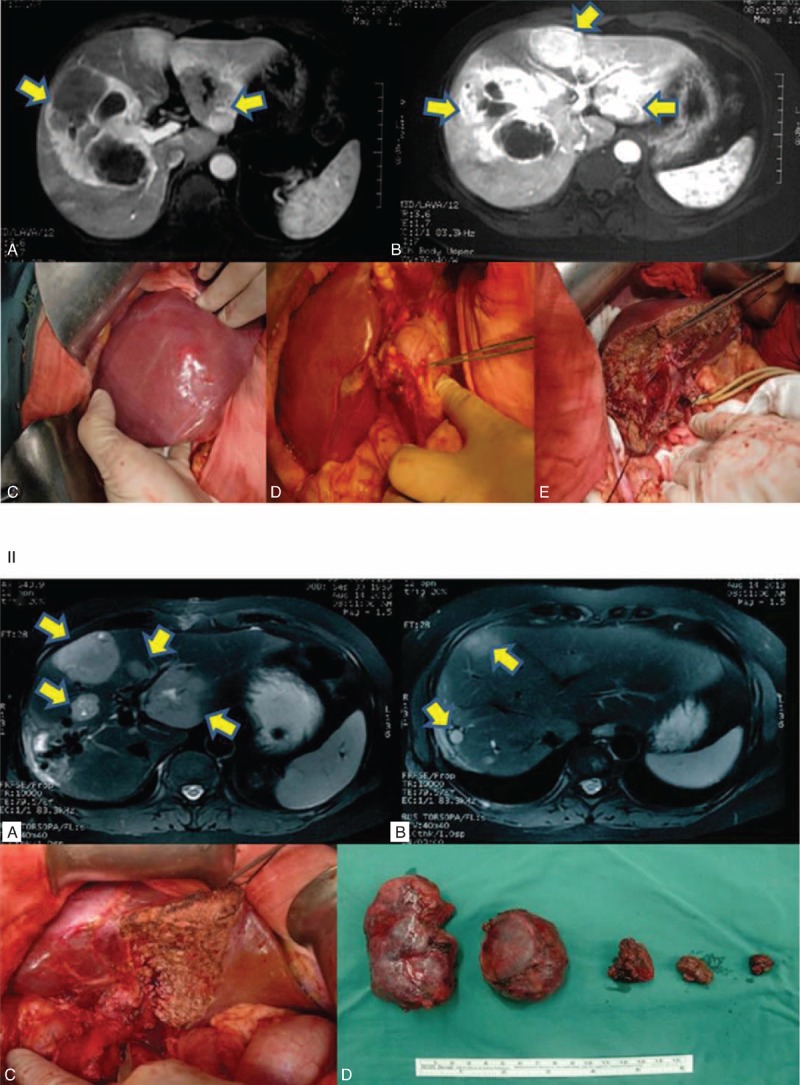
MRI and surgical data on 1 patient with G2-level NELM. A 52-year-old female patient was diagnosed with NELM with 6 liver neoplasms totaling 31 cm in size. Two separate surgical resections were performed. The first resection removed the largest tumor of 15 cm. The liver was allowed to regenerate to sufficient volume to sustain secondary resection. All 5 other neoplasms were removed during the secondary resection, which was performed 4 months after the first one. The patient was in good condition at the time of the preparation of this manuscript. Panel I, MRI images and key steps of first resection. (A & B) MRI images, arrows indicate locations of tumors; (C) a 15-cm tumor was found that covers sections of V, VI, VII, and VIII of liver; (D) primary tumor was found on the lesser curvature of the stomach; (E) remnant tissue of right lobe after resection. Panel II, MRI images and photos of second resection. (A & B) MRI images, arrows indicate locations of tumors; (C) remnant tissue after resection; (D) tumors resected from second operation. MRI = magnetic resonance imaging, NELM = neuroendocrine liver metastases
